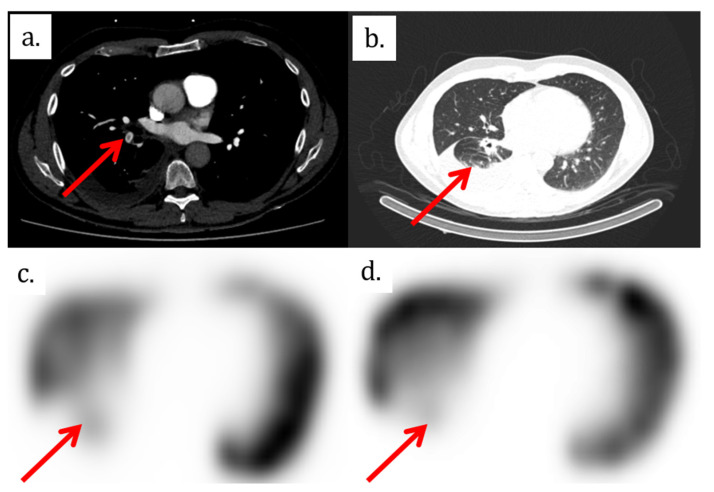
(a) Axial view of CTPA shows a segmental filling defect in a segmental artery of the right lower lobe. (b) Lung CT images show retractile neoplastic lesion of the right inferior lobe with a low right pleural effusion. (c) and (d) show perfusion and ventilation SPECT images, respectively, with a matched defect in the right lower lobe.
